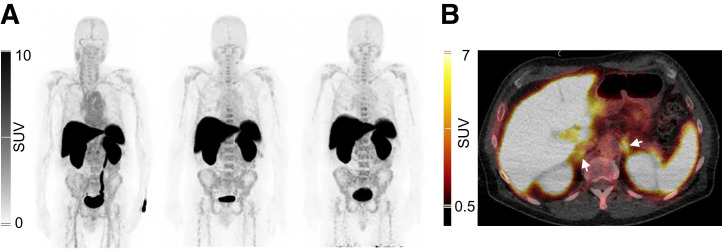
Normal biodistribution of [^68^Ga]Ga-NOTA-anti-CD206-sdAb. (A) Anterior maximum intensity projections of patient 1 at 11 min (left), 90 min (center), and 150 min (right) after injection. (B) Axial PET/CT fusion image at 90 min after injection, showing uptake in liver, spleen, kidneys, and adrenals (arrows). No tracer uptake is visible in lung lesion of this patient.
